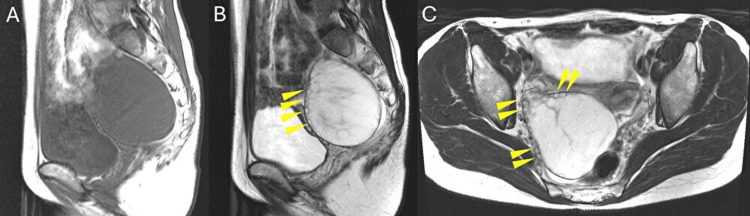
Pelvic MRI findings. (A) T1-weighted sagittal image. (B) T2-weighted sagittal image. (C) T2-weighted axial image. The enlarged right ovary measures 77.9 × 68.7 × 81.0 mm and contains multiple peripheral ovarian follicles. The lesion is isointense on T1-weighted imaging and hyperintense on T2-weighted imaging, consistent with edematous stroma. Arrowheads indicate the small cystic structures.
